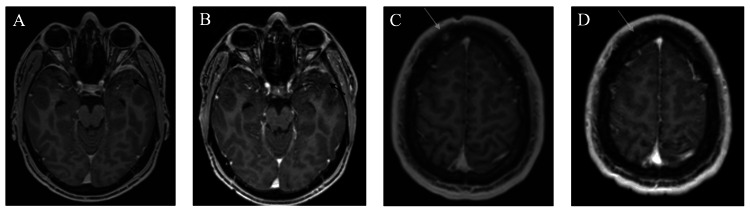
MRI brain findings after four cycles of cladribine. (A) Interval decrease in size of left sphenoid mass, followed by (B) stable size; (C) interval decrease in size of the right frontal calvarium lesion (arrow), followed by (D) stable size (arrow). MRI, magnetic resonance imaging
